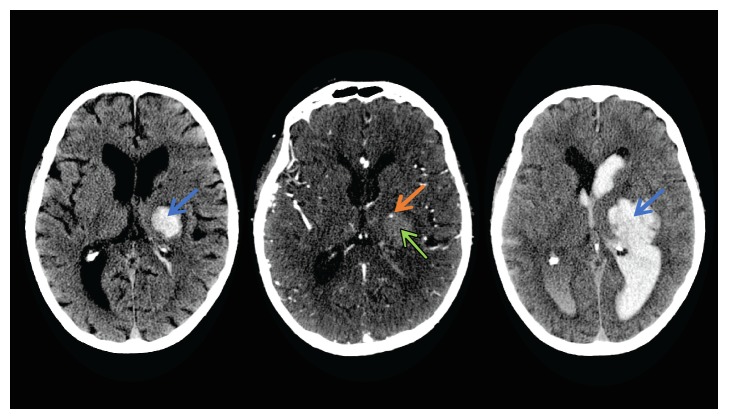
These images depict a left hemisphere ICH. A single sign with a spot-like appearance on CTA (orange arrow). The hyperdense area (blue arrow) in the first picture signifies an early hematoma in a baseline noncontrast CT. The second image shows that a spot sign (orange arrow) within the hematoma (green arrow) is discontinuous to any outside vessels and has a higher CT HU than the background hematoma in real CTA. The third image shows an expanding hematoma (blue arrow) in a 24 h follow-up noncontrast CT. Image provided by Dr. Andrew M. Demchuk, M.D., FRCPC.
